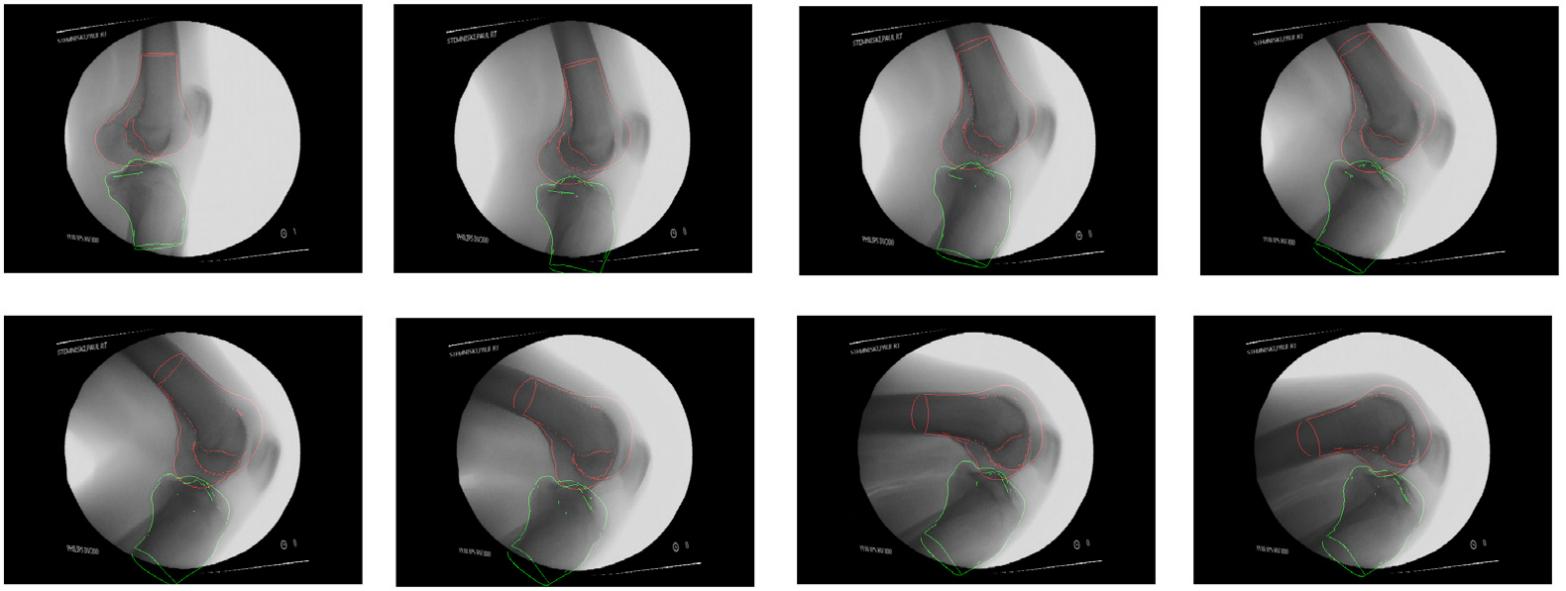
3-D model edge overlay on the x-ray images in key poses for femur and tibia during DKB (subject 4).
